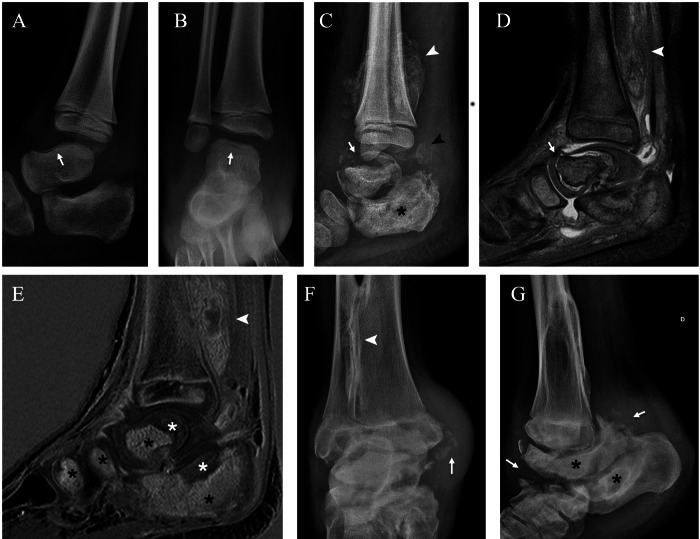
Neuropathic arthropathy of the right ankle in a boy with hereditary sensory and autonomic neuropathy type IV. Lateral (A) and anteroposterior (AP) (B) radiographs at 6 years of age show a linear cortical fracture of the talus (arrows). Lateral radiograph 1 month after the first set of radiographs (C) shows worsening of talar fractures (arrow), sclerotic and lytic changes of the calcaneus (asterisk) and loose bone fragments (black arrowhead); note the exuberant callus due to a fibular fracture (white arrowhead). Sagittal proton density (PD) (D) and T1 post-gadolinium MRI (E) show the talar fractures delineated by the fluid-filled cleft on the articular surface (arrow) and a diffuse enhancement of tarsal bones (black asterisk), respectively, in contrast to non-enhanced subchondral areas of sclerosis (white asterisk). The fibular callus is evident behind the tibia with increased enhancement (white arrowhead). AP (F) and lateral (G) radiographs at 14 years of age show permanent deformity with collapse of the tibio-talar and talo-calcaneal joints, talar and calcaneal sclerosis and flattening (asterisk), loose bone fragments (arrow) and tibio-fibular fusion (arrowhead)
